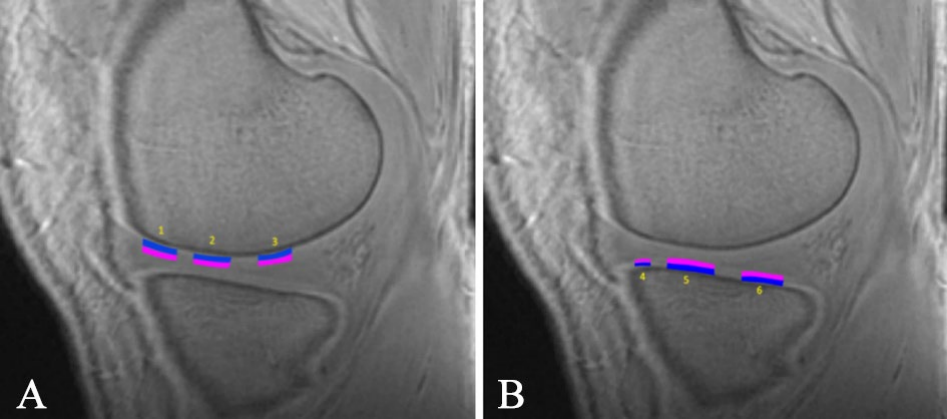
Cartilage position measured in this study. Zero TE MR imaging demonstrates the cartilage at the femur (A) and tibia (B) in the knee joint. The marked targets were measured. 1, The femur cartilage at the anterior horn of the medial (lateral) meniscus. 2, The cartilage at the load-bearing region of the medial (lateral) condyle of the femur. 3, The femur cartilage at the posterior horn of the medial (lateral) meniscus. 4, The tibia cartilage at the anterior horn of the medial (lateral) meniscus. 5, The cartilage at the load-bearing region of the medial (lateral) condyle of the tibia. 6, The tibia cartilage at the posterior horn of the medial (lateral) meniscus. The blue rectangle indicates the deep layer of cartilage, and the pink rectangle indicates the shallow layer of cartilage.
